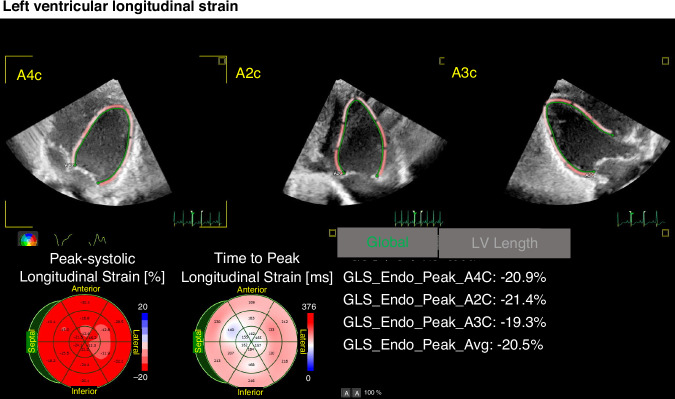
Analysis of LV longitudinal strain by speckle-tracking echocardiography. *A4C* apical four-chamber view, *A2C* apical two-chamber view, *A3C* apical three-chamber view*, GLS* global longitudinal strain*, LV* left ventricular, *Endo* endocardial*, Avg* average of A4, A2C, andA3C.
